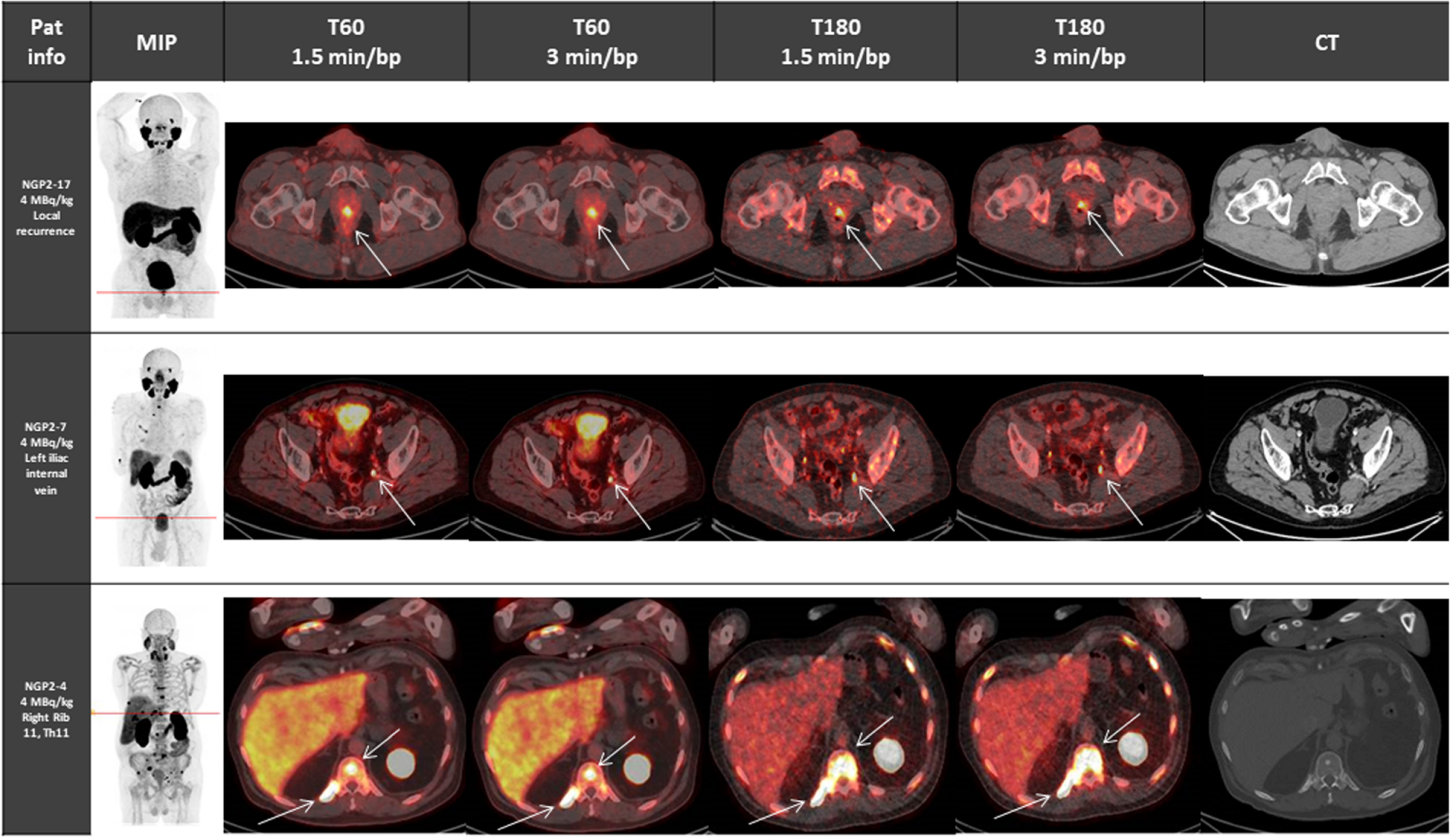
Overview of patient images who were administered 4 MBq/kg who presented with common lesion locations: local recurrence (first row), lymph node metastases (middle row) and bone metastases (last row), each presented as a MIP image (at T60, 3 min/bp) followed by axial images (axis line displayed on MIP image) of all reconstructed time frames (T60, 1.5 and 3 min/bp and T180, 1.5 and 3 min/bp), and the high dose CT image
